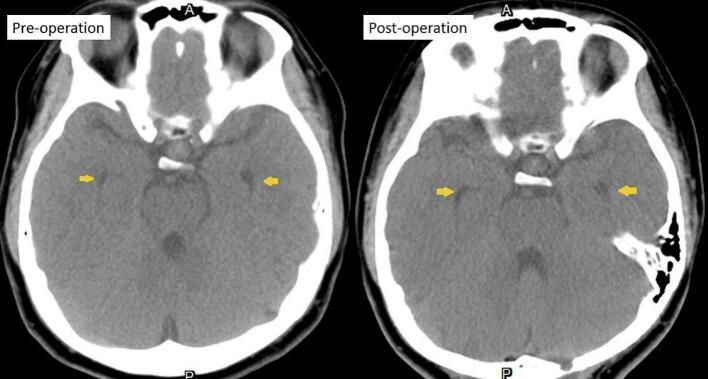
indicates that there is no statistically significant difference in the dimensions of the bilateral ventricular temporal horns when comparing preoperative and postoperative brain computed tomography images.
